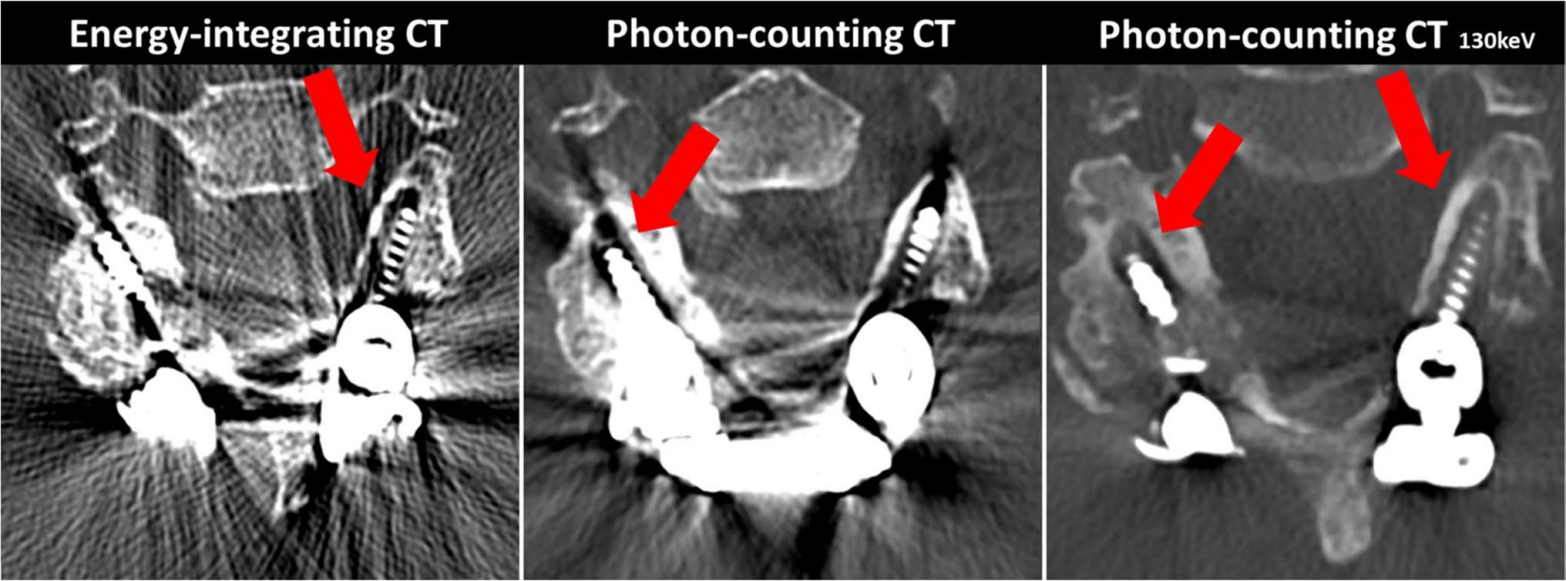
Image example of an 85-year-old patient with post-surgical CT after spinal fusion due to traumatic fracture and suspected screw loosening (window center 500 HU, window width 2000 HU). Images depict an axial slice at the height of the 5th cervical vertebra. EID-CT shows severe artifacts caused by metallic implants resulting in a substantially impaired image quality and reduced diagnostic confidence. Screw loosening was suspected in the left pedicle (arrow). After treatment of a delayed wound healing, follow-up CT imaging was performed on a PC-CT after interim external immobilization of the neck. PC-CT_std_ shows significantly reduced artifacts and better depiction of the bone resorption and also reveals loosening of the right screw (arrow). PT-CT_130 keV_ reconstruction shows almost no artifacts and clearly depicts the bone resorption around both screws (arrows). EID-CT = energy-integrating CT; PC-CT_std_ = photon-counting CT; PT-CT_130 keV_ PC-CT with monoenergetic reconstructions at 130 keV
